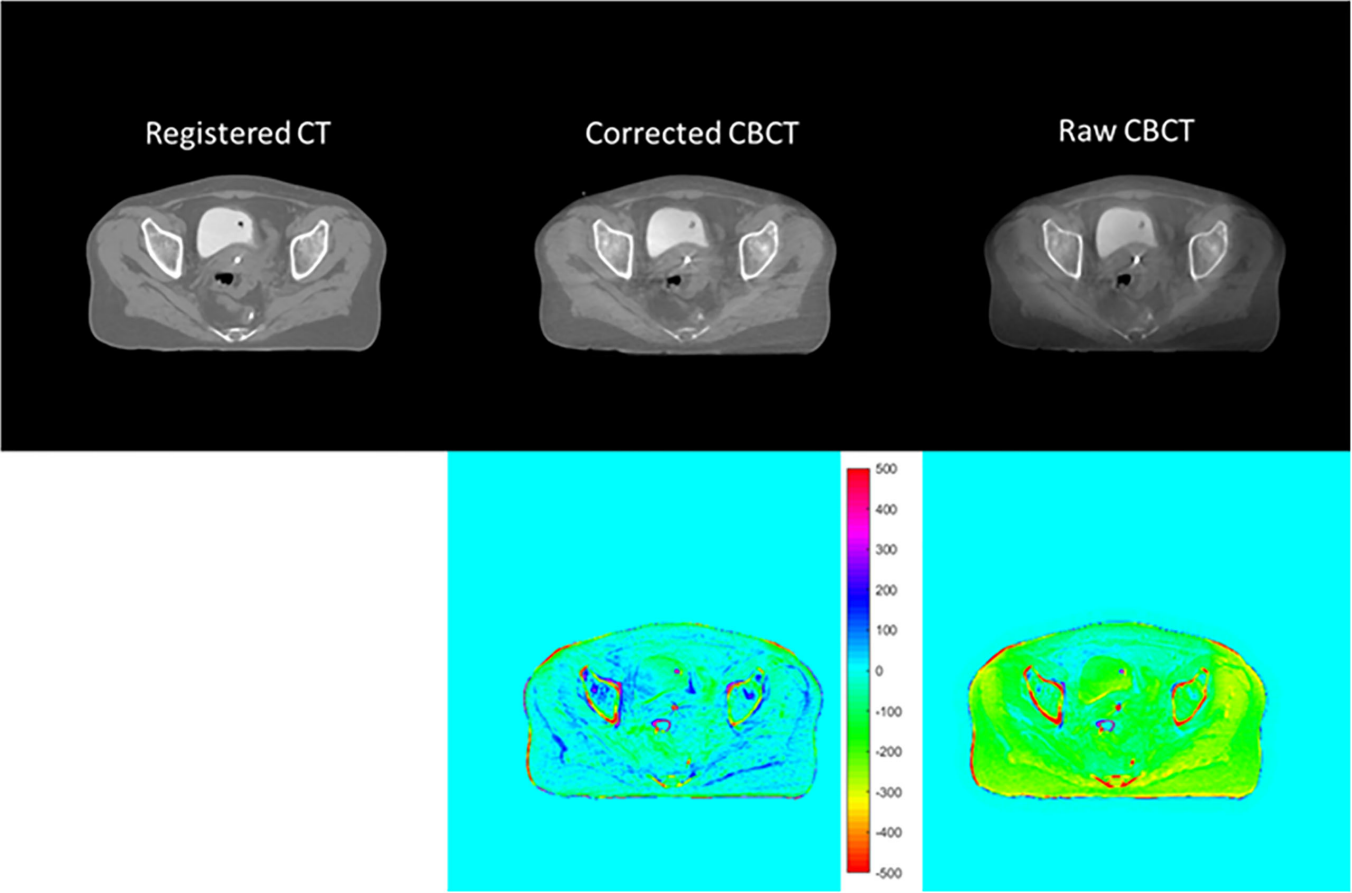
CT/CBCT images of the same patient in Figure 6 in the axial view and the CT number difference map of CBCT and CT; display window: [−500 500] HU.
